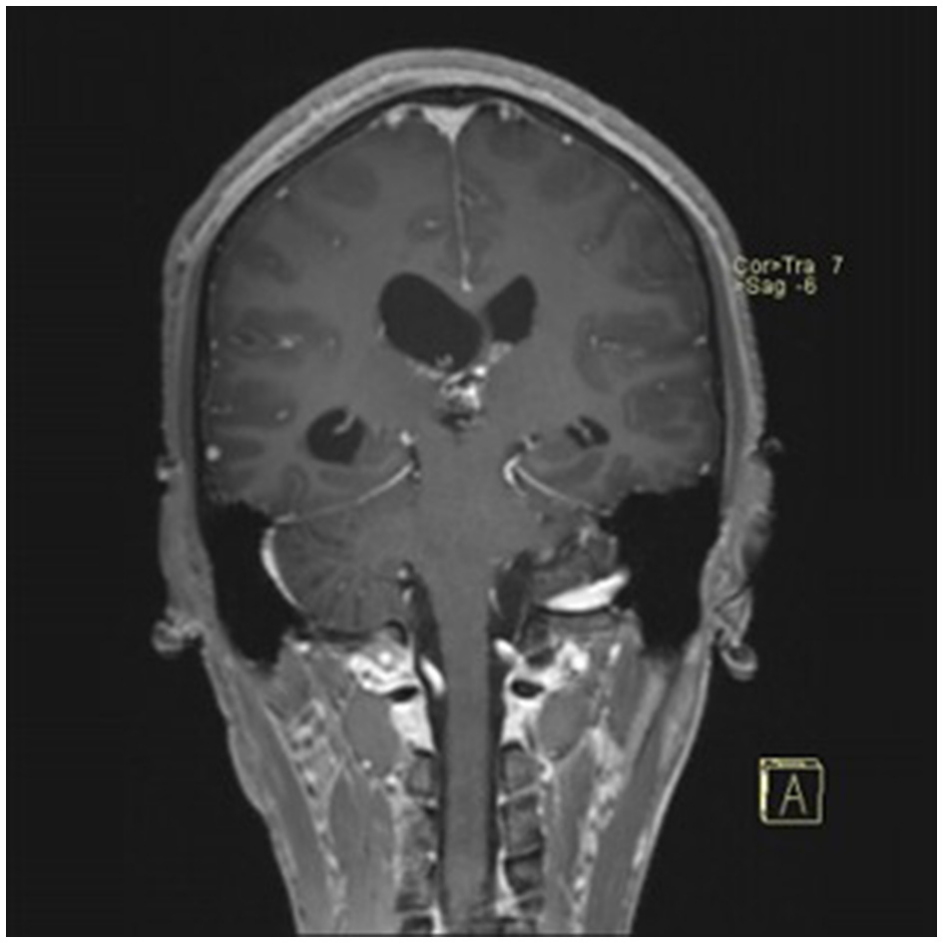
Postoperative contrast-enhanced MRI (coronal view) showing no residual tumor.
